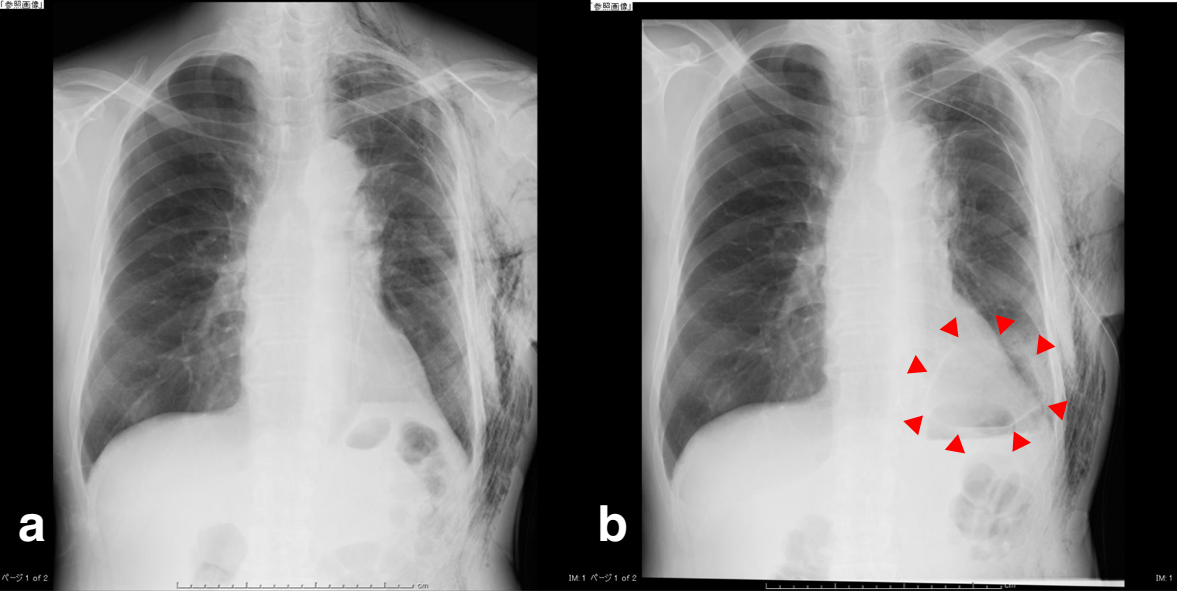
Postoperative imaging. a Chest X-ray on POD7 reveals no dead space in the left lung field. b Chest X-ray on POD12 reveals a cavity in the left lower lung field (red arrowheads)
